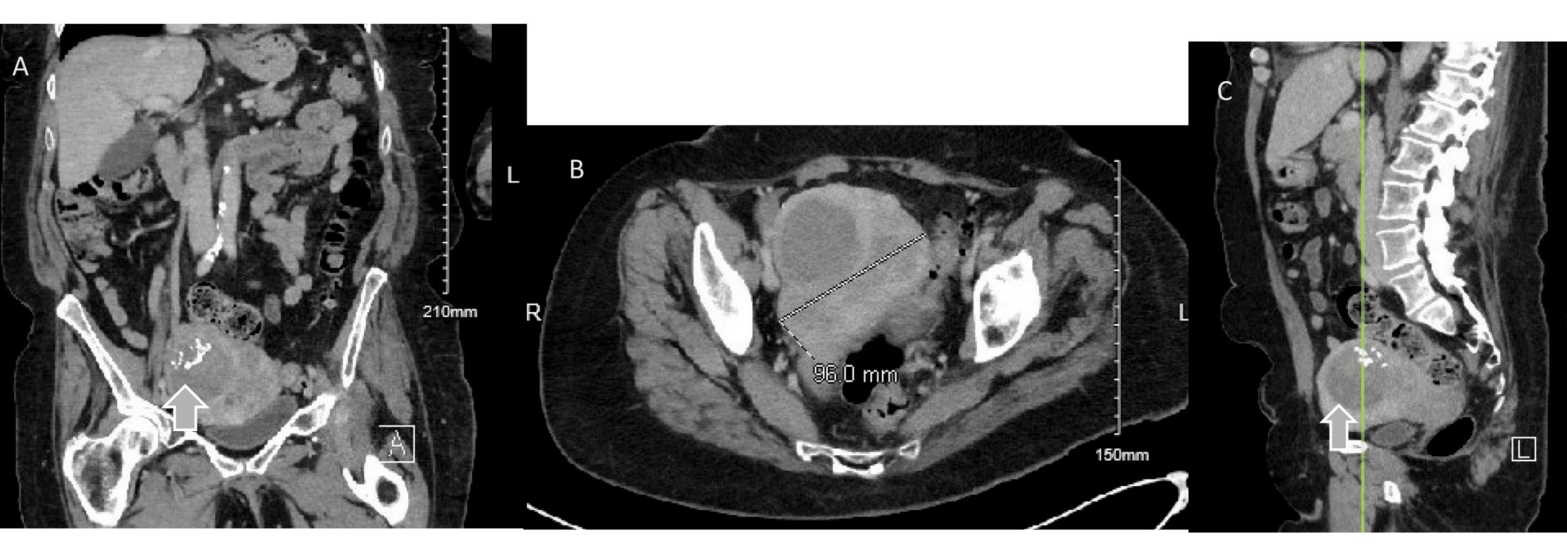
A CT scan of the chest, abdomen and pelvis (A) coronal, B) axial, and C) sagittal images) revealed the uterus to be significantly enlarged (measured at 11x9.6 cm) with multiple areas of cystic and calcific foci, presumably leiomyomatous enlargement.
